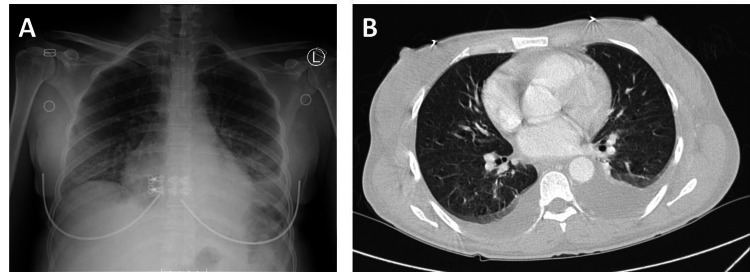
Radiographic studies of the patient (A) Chest x-ray shows hazy opacity at the lung bases compatible with effusions. (B) CT thorax demonstrates bilateral pleural effusions, left greater than right, with adjacent passive atelectasis in the lower lobes.
